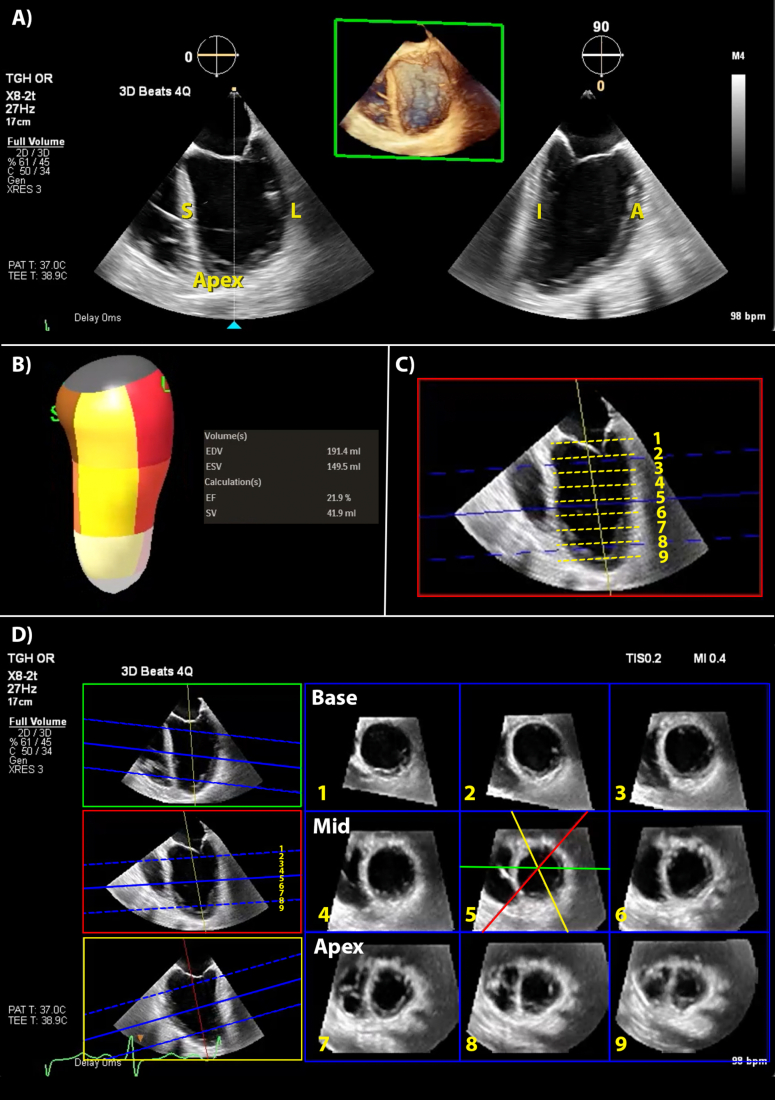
Three-dimensional transoesophageal echocardiography views of the left ventricle. (A) This is a full volume acquisition of the LV using multi-beat acquisition over 4-beats at a frame rate of 27 Hz. (B) This shows a 3D volumetric model of the LV, from tracking of the endocardial border across the cardiac cycle. Myocardial segments are colour coded. Calculation of EF without geometric assumptions occurs from this dataset. (C) Reconstruction of the left ventricular full volume acquisition can show multiple short axis planes across the ventricle. This aids in identification of RWMA. The planes, numbered 1–9, correspond to those seen in the machine display shown in part (D). (D) Multiple short-axis slices of the LV reconstructed from the full volume dataset. Three MPR planes seen in green, red and yellow planes on the left are aligned across the left ventricular cavity. Aligning the blue dotted lines between the base and apex delineates the position of the first and last short axis slices seen on the right. The addition of numbers aids understanding when viewed with part (C). A, anterior wall of left ventricle; EDV, end-diastolic volume; EF, ejection fraction; ESV, end-systolic volume; I, inferior wall of left ventricle; L, lateral wall of left ventricle; LV, left ventricle; RWMA, regional wall motion abnormalities S, intraventricular septum; SV, stroke volume.
